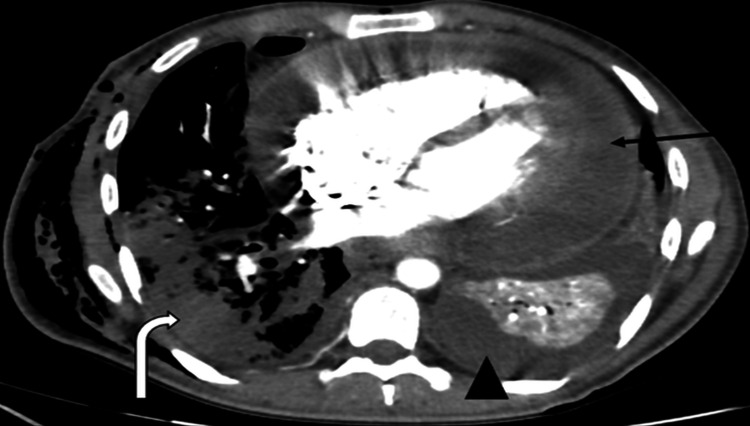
Pericardial tuberculosis ADA: adenosine deaminase; CT: computed tomography Axial contrast-enhanced thoracic CT angiography showing pericardial effusion with associated pericardial enhancement (black arrow), pleural effusion (arrowhead), and consolidations in the right lung parenchyma (curved arrow). Diagnosis confirmed by analysis of pericardial fluid, revealing high ADA levels and positive culture for *Mycobacterium tuberculosis*
